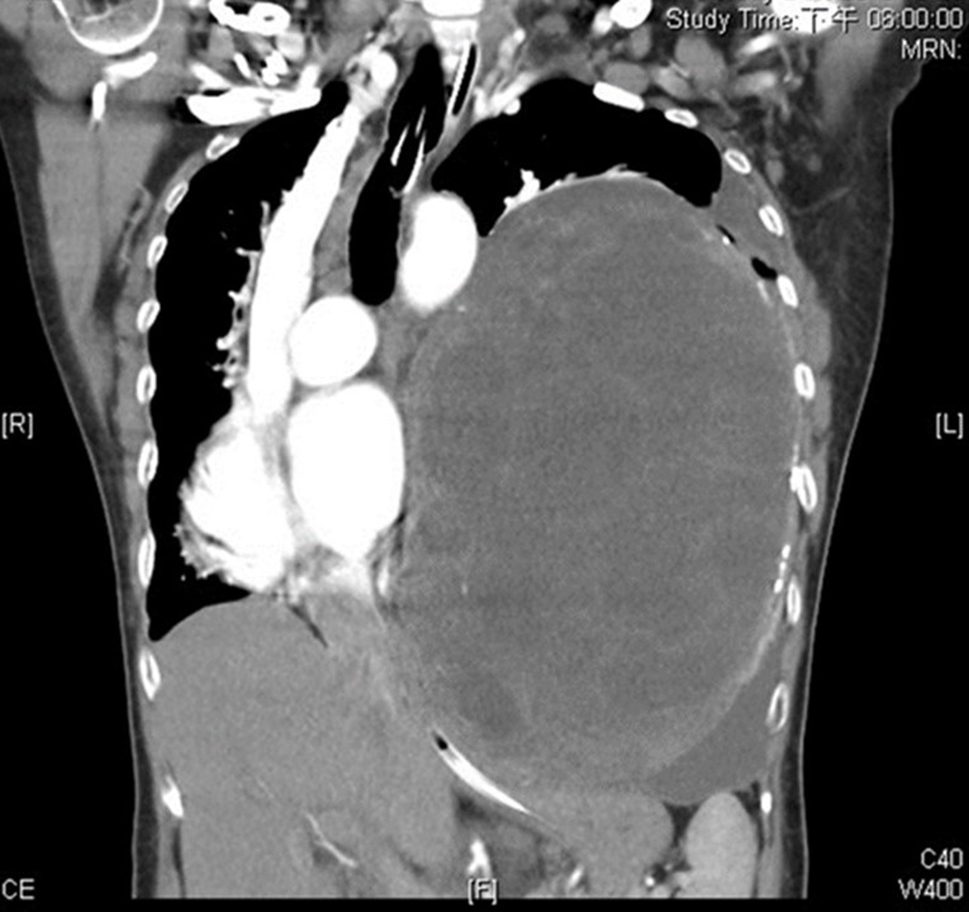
Chest computed tomography showed huge tumor causing heart compression
